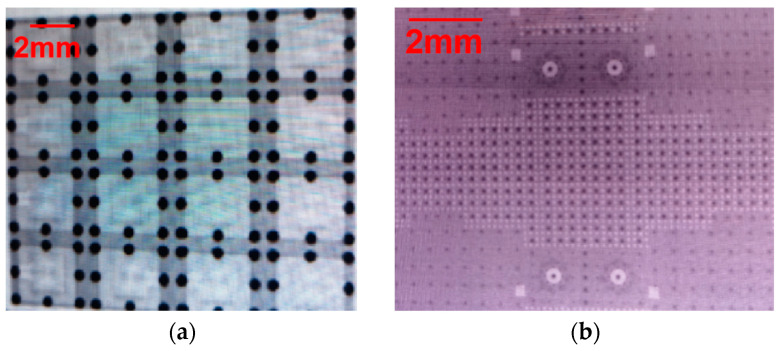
X-ray images of (a) an interconnection between glass micro substrates and (b) an interconnection between glass micro substrates and silicon micro substrates.
